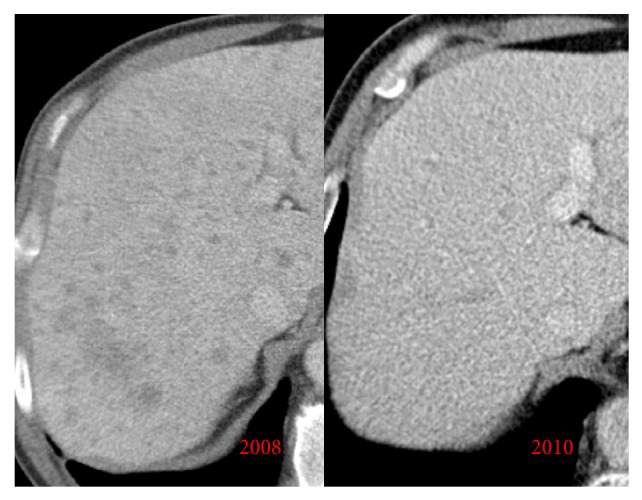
Axial contrast-enhanced abdominal CT images: comparison of CT abdominal images from 2008 and 2010 after chemotherapy, demonstrating resolution of the liver nodules, common in acute liver involvement of LCH.
